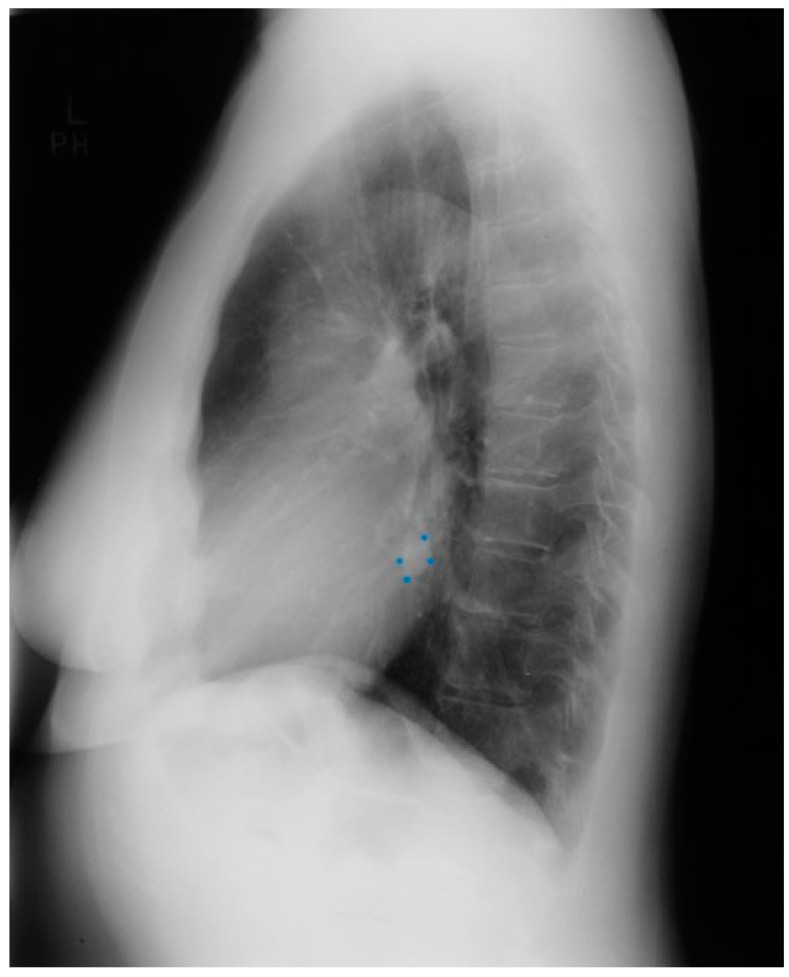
Lateral chest X-ray of persistent left superior vena cava: This is the lateral chest X-ray of the same patient with persistent left superior vena cava (PLSVC), whose images are discussed above. The most noteworthy finding is the round density located close to the posterior cardiac border, which is about equidistant from the leaves of the diaphragm and the radiolucent left main bronchus. This density (blue dots) is in the region of the posterior atrioventricular groove, where the coronary sinus is located. The coronary sinus is enlarged because, in addition to carrying coronary venous blood, it is carrying venous blood from the upper body, including the arms, head and neck, and chest. Thus, the posteroanterior and lateral chest X-rray can demonstrate findings that identify PLSVC. The anteroposterior chest X-ray done after coronary artery bypass graft surgery demonstrated passage of the right internal jugular venous introducer to the left side of the upper chest. The pulmonary artery catheter threaded through this introducer entered the PLSVC, then the coronary sinus to the right atrium, followed by the right ventricle and out to the pulmonary trunk. The patient recovered well from her bypass surgery.
